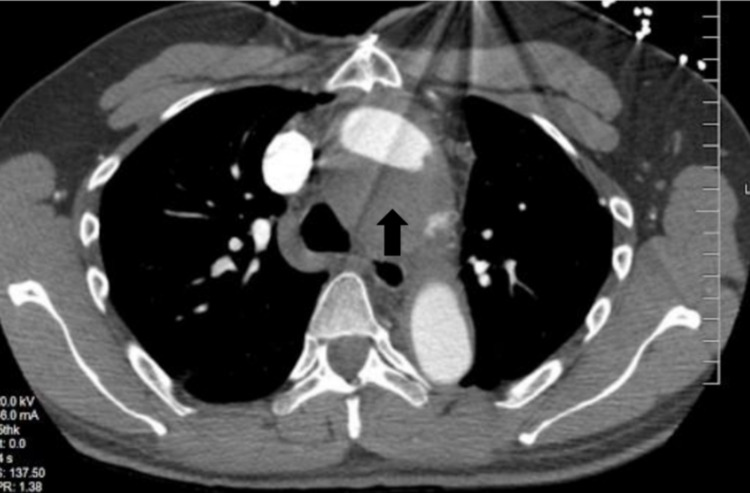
CT angiogram of the chest (axial view) Note the pseudoaneurysm inferior to true aortic lumen with the arrow pointing toward the thrombosed component.
